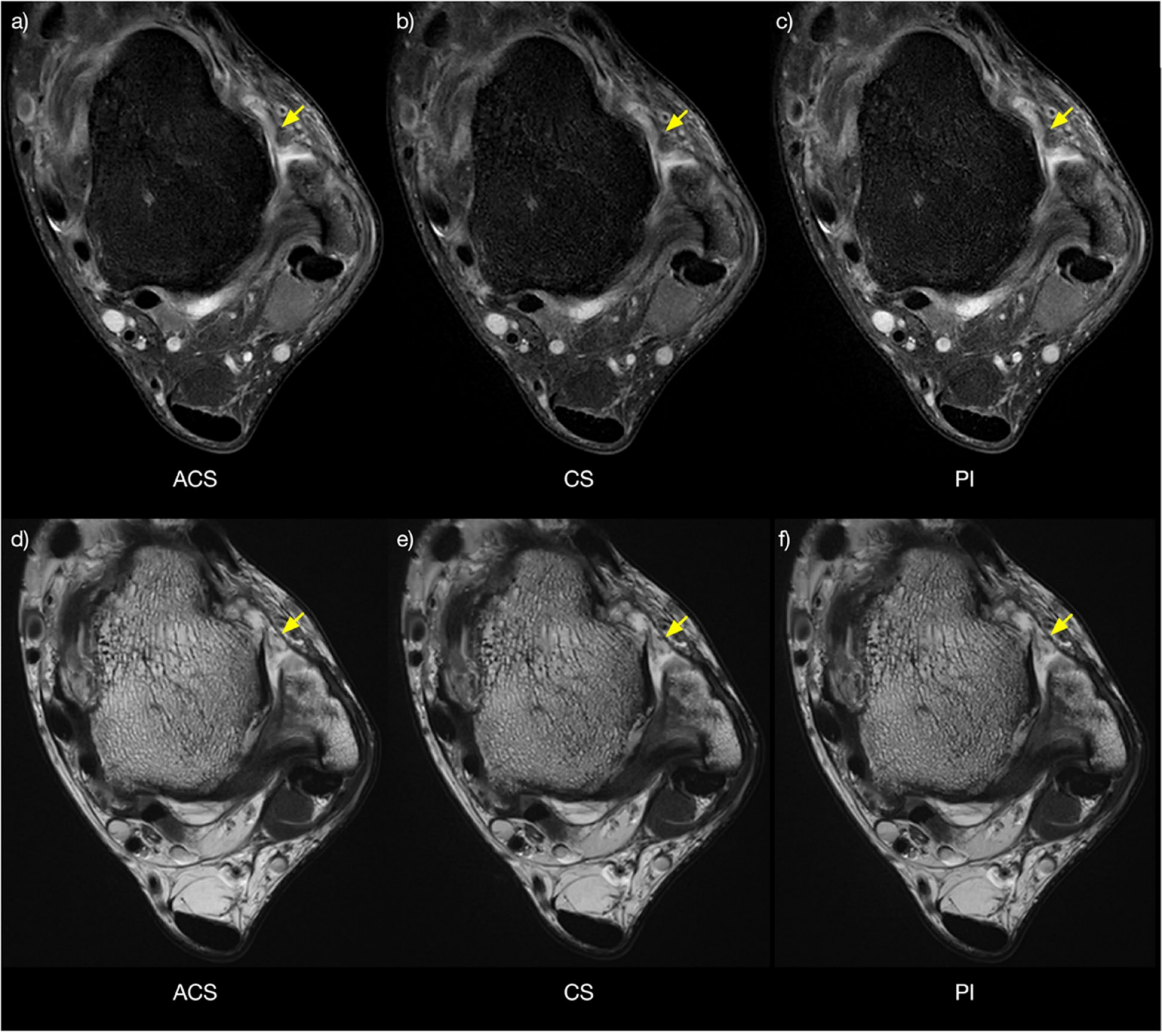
Axial PD-weighted fast-sat images acquired using ACS (a), CS (b), and PI (c) and axial T2-weighted images acquired using ACS (d), CS (e), and PI (f) showing the ankle of a 34-year-old male patient with anterior talofibular ligament rupture (arrows). *ACS* Artificial intelligence-assisted compressed sensing, *CS* Compressed sensing, *PD* Proton density, *PI* Parallel imaging
